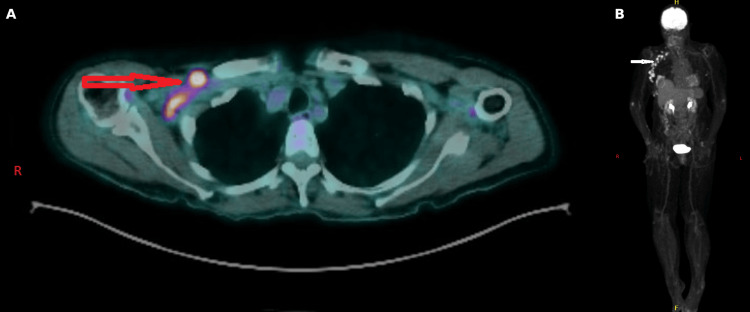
PET-CT images showing fluorodeoxyglucose (FDG)-avid right axillary lymph nodes on (A) fused axial imaging and (B) whole-body maximum intensity projection imaging.
